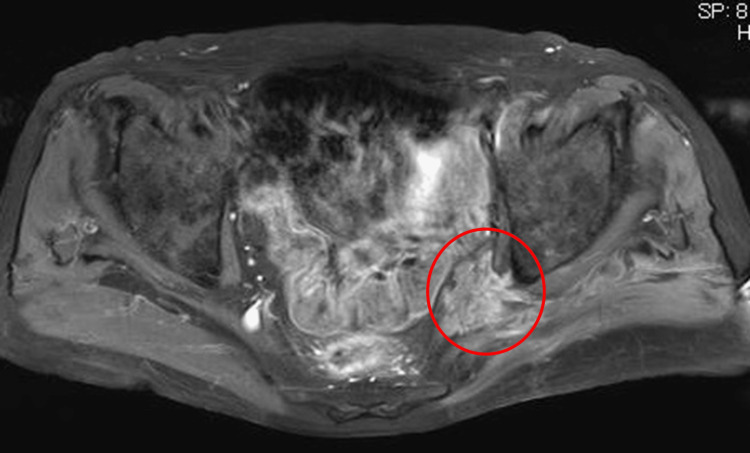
Pelvic enhanced magnetic resonance imaging Enhanced magnetic resonance imaging (MRI) shows a mass with indistinct borders and homogeneous contrast in the region of the left greater sciatic foramen.
